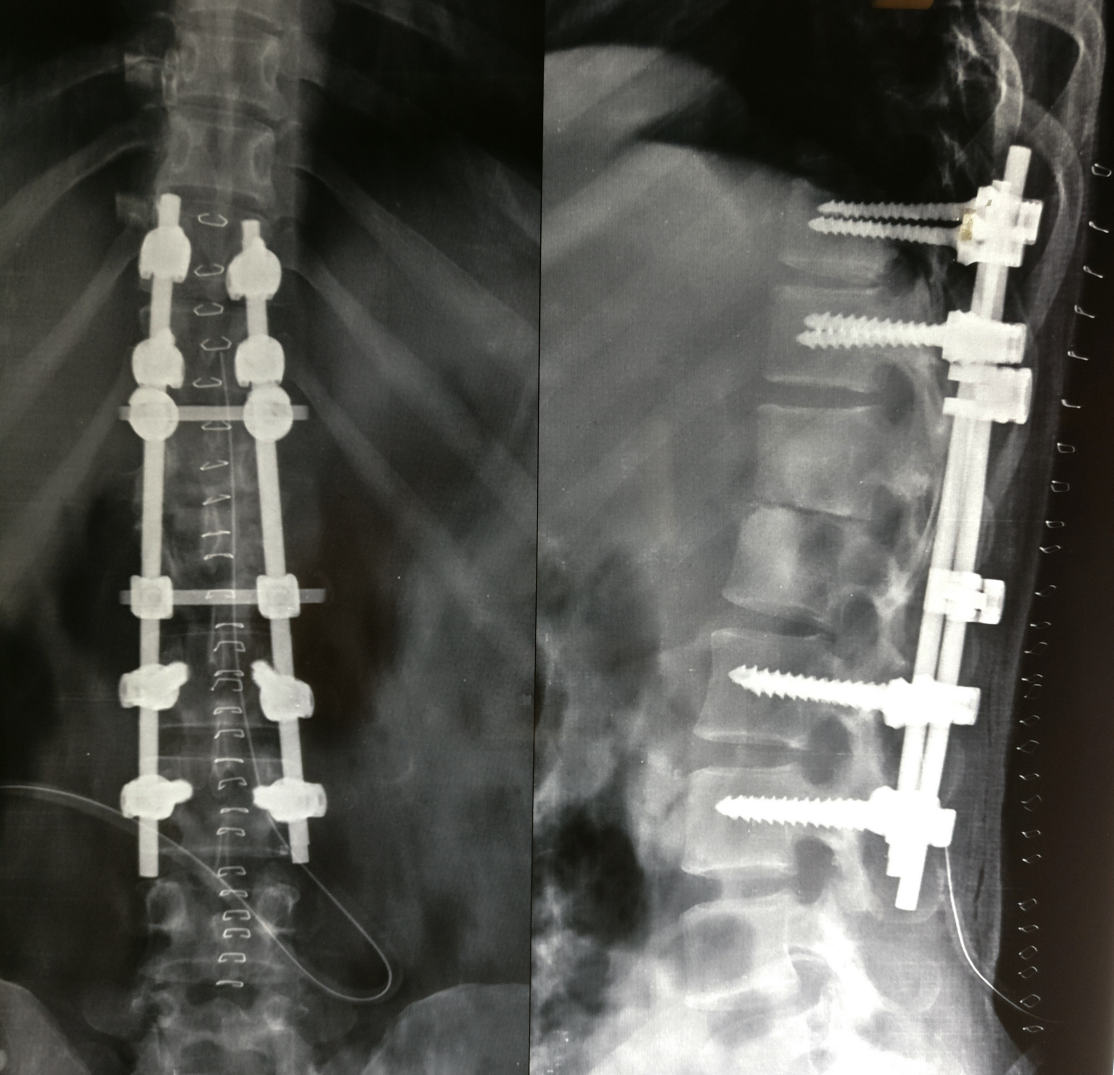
Post-op radiograph of dorso-lumbar spine (antero-posterior and lateral views) Posterior fixation with pedicle screws and rods with two interlinks showing correction of kyphotic deformity.
